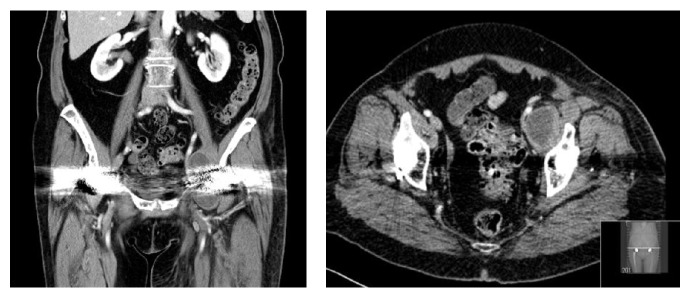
CT angiography showing a large cystic mass compressing the left iliac vessels.
